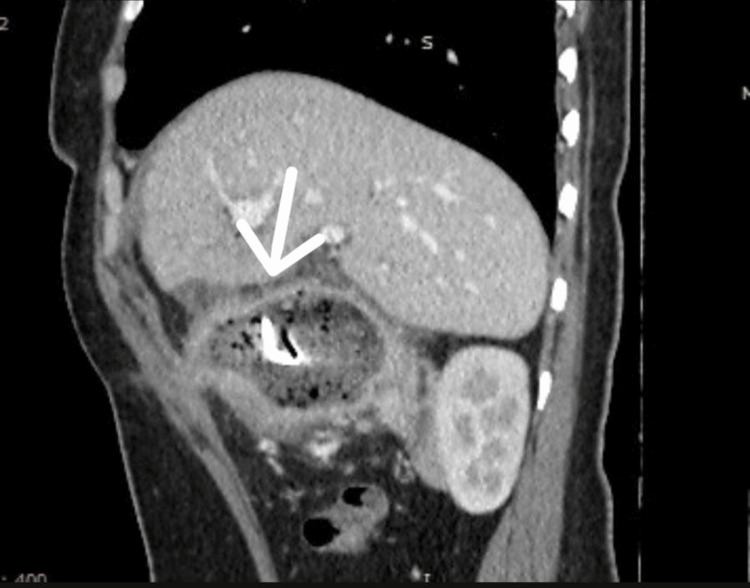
CT (sagittal view) of the abdomen and pelvis showing the retained surgical pack (arrow)
